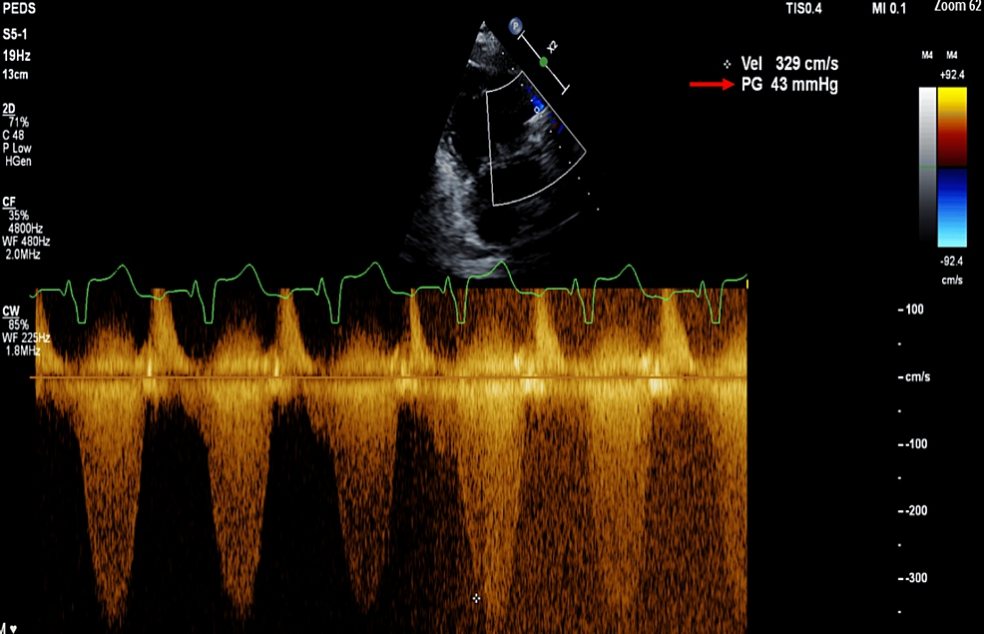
Transthoracic echocardiogram performed on January 24, 2023, indicating a reduction in the pressure gradient across the right ventricular outflow tract with an improved value of 43 mmHg.
